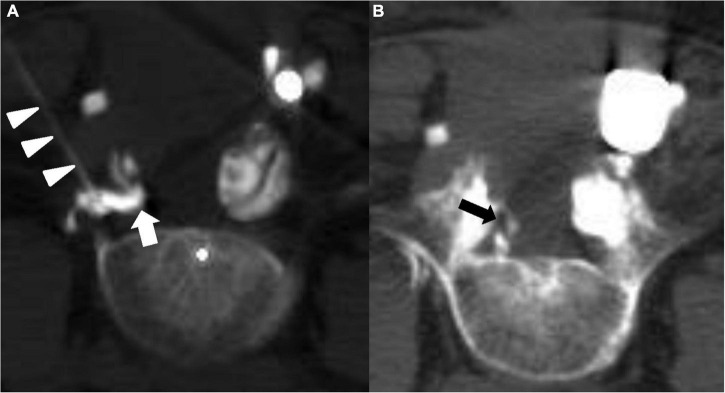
CT-guided transforaminal injection at the level of L5-S1. Axial CT for the extent of contrast spread. (A) Visualization of the needle in the epidural space (arrowheads) and the dye (thick arrow) blocked by the scar. (B) Contrast filling around the right S1 root (thick arrow) at the ventral epidural space.
